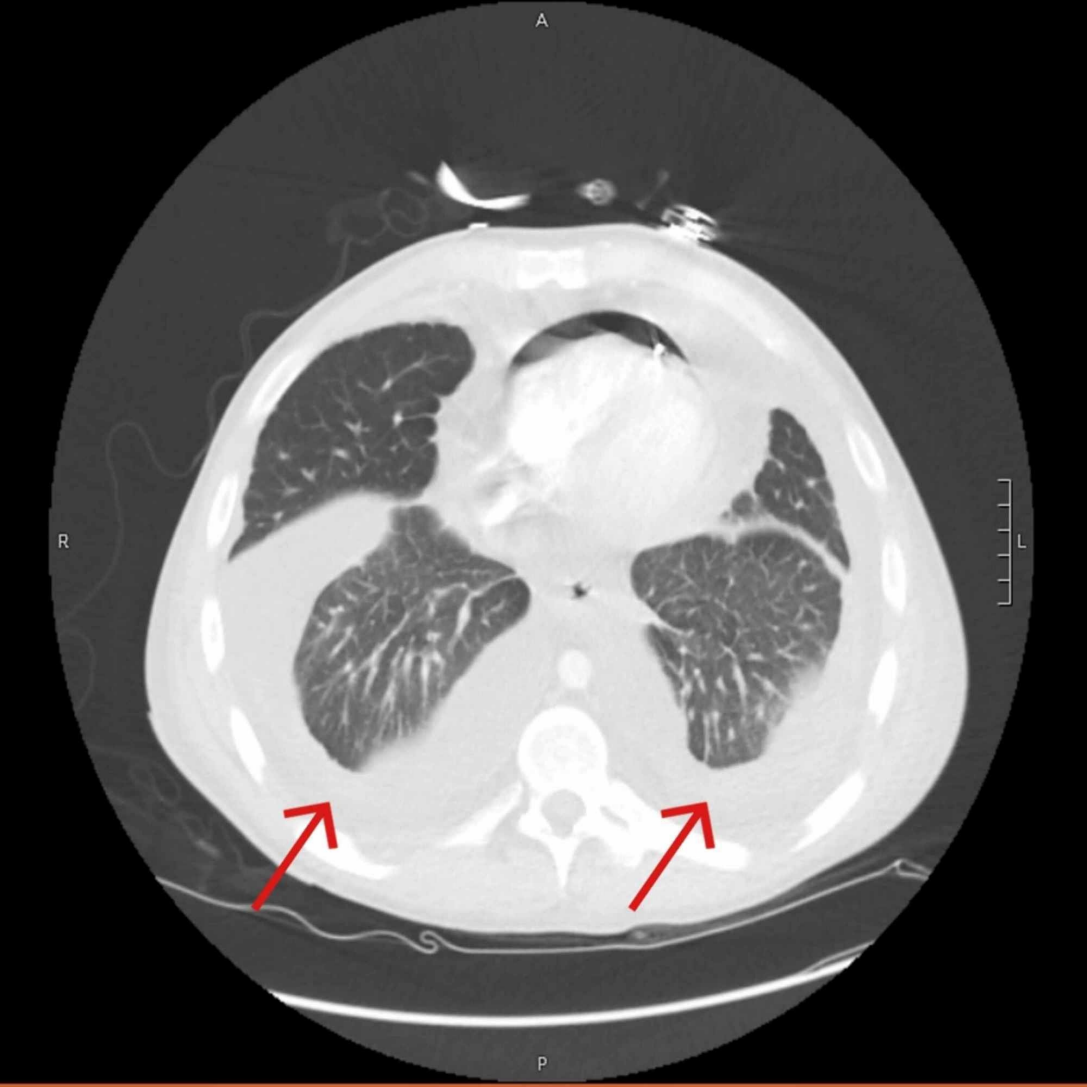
Chest CT showing bilateral pleural effusions
